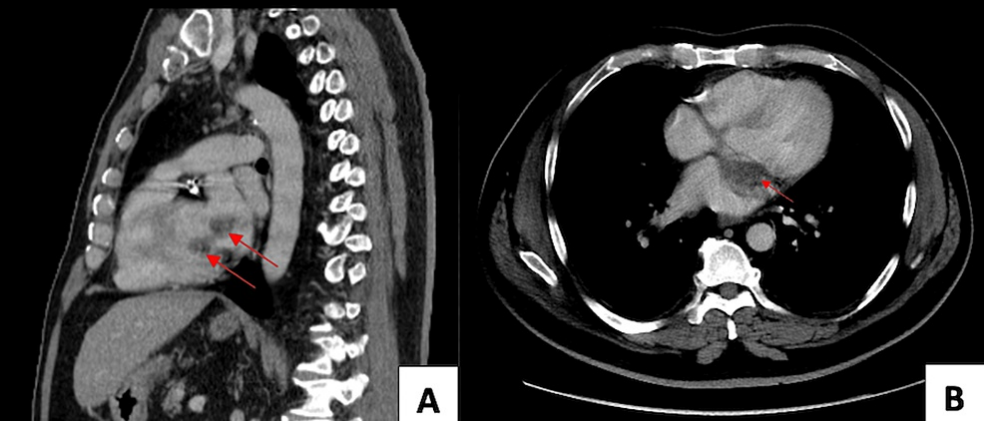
Transverse (A) and axial (B) CT scans identifying a low-attenuating lobular region in the left atrium and left ventricle, centered at the left mitral valve, and measuring 2.3x3.5 cm (red arrows). CT: computed tomography
